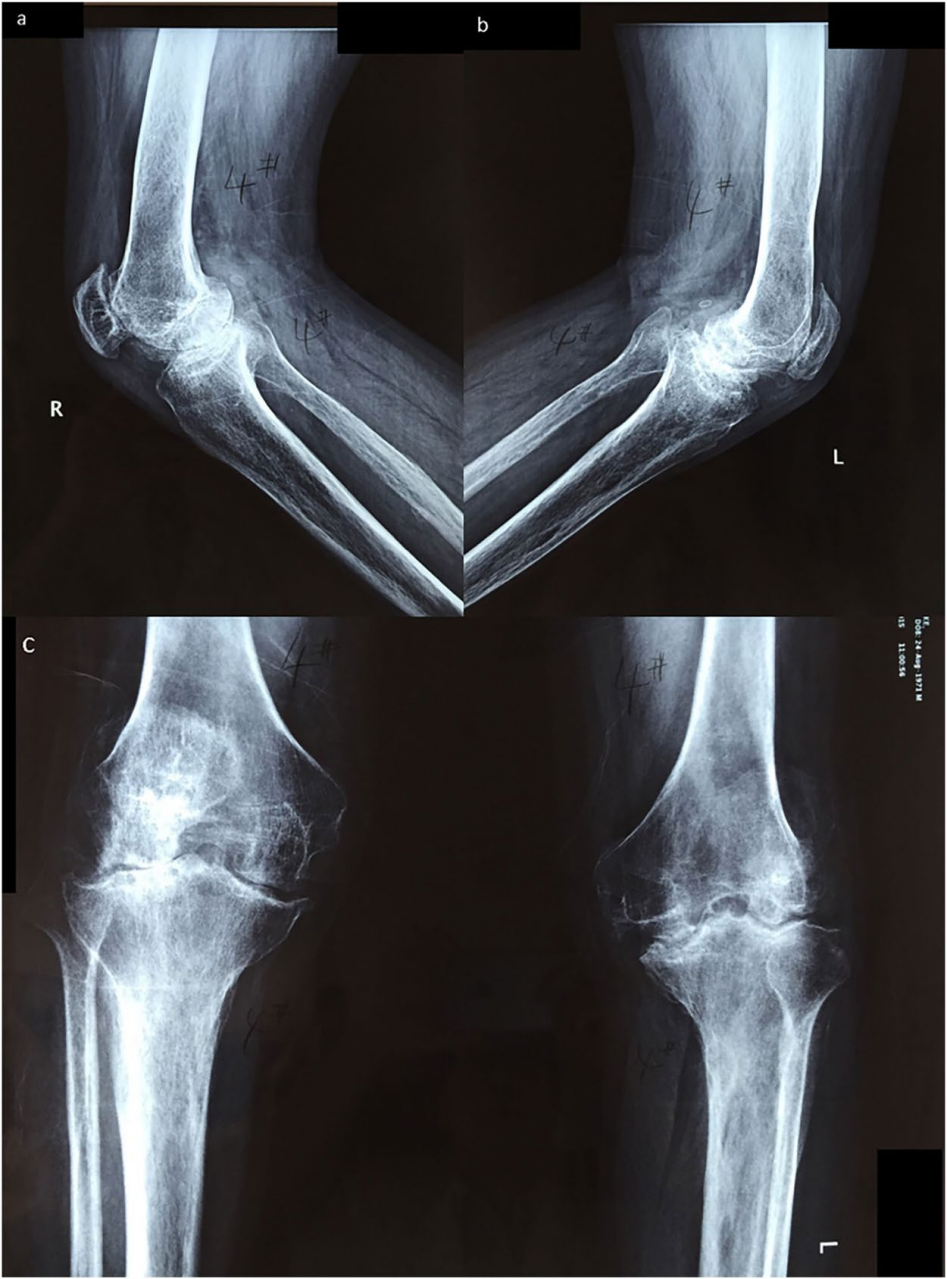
Preoperative X-ray image of the same patient presented in Fig. 1. The film indicated end-stage hemophilic arthropathy in both knee joints. (a) Lateral view of the right knee joint. (b) Lateral view of the left knee joint. (c) Anteroposterior view of both knee joints. Patient information is masked to protect the patient’s privacy.
